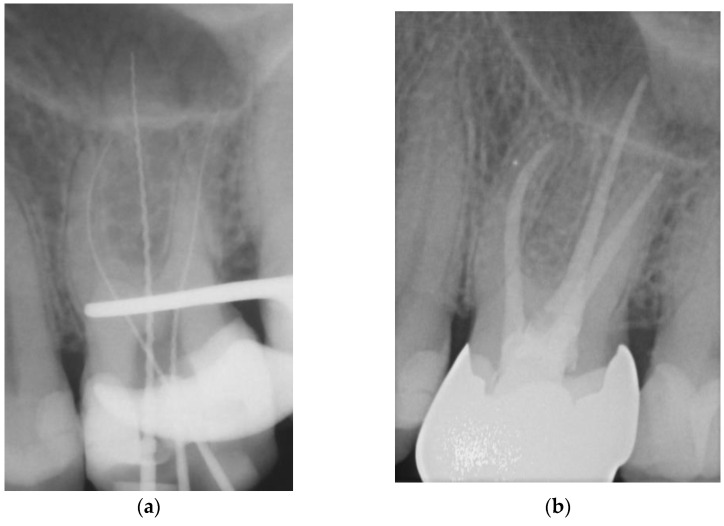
Representative example of root canal filling using the single-cone obturation technique with gutta-percha and AH Plus^®^ (SCGP); working length radiograph of tooth 26 (a); 7-year follow-up radiograph showing complete apical healing (b).
